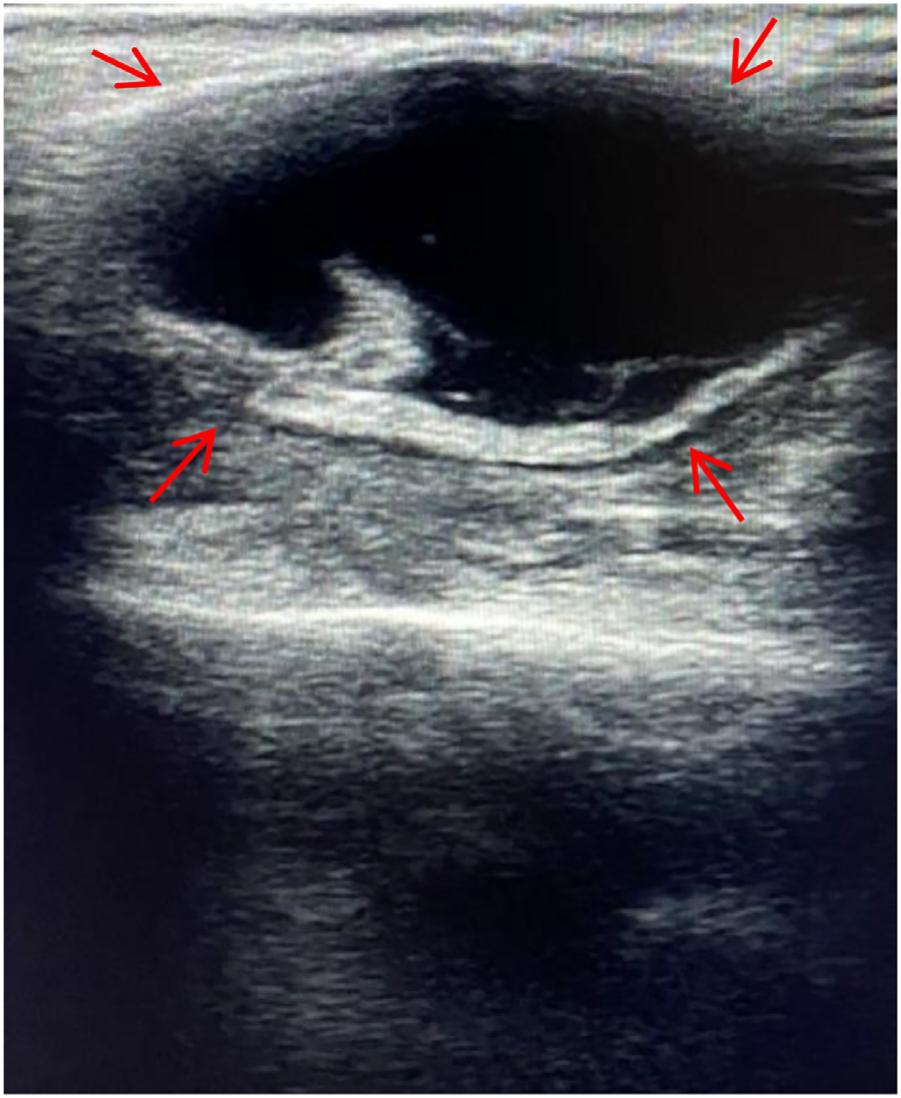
Ultrasonography of the right parotid gland showing a well-defined anechoic lesion in the superficial lobe (indicated by red arrows). No significant vascularity is noted within the lesion on Doppler study.
